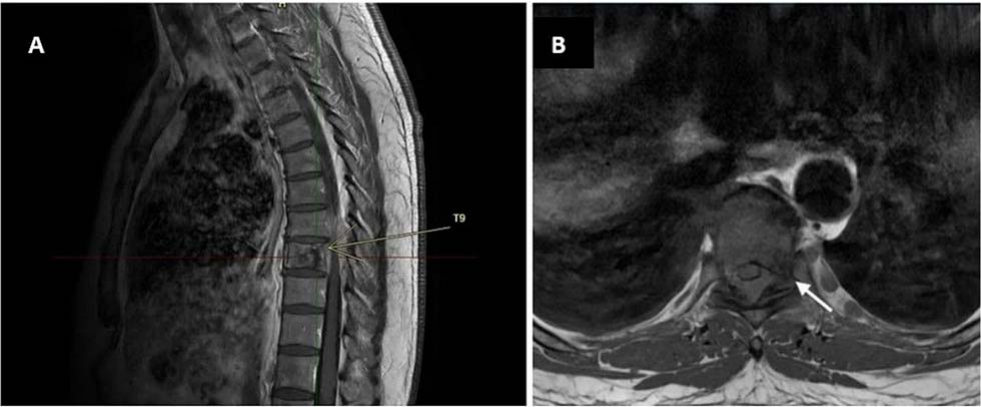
Figure 1 Contrast-enhanced MRI of the thoracic spine. Sagittal view (A, yellow arrow) and axial view (B, white arrow) show an abnormal signal in the T9 vertebra.
